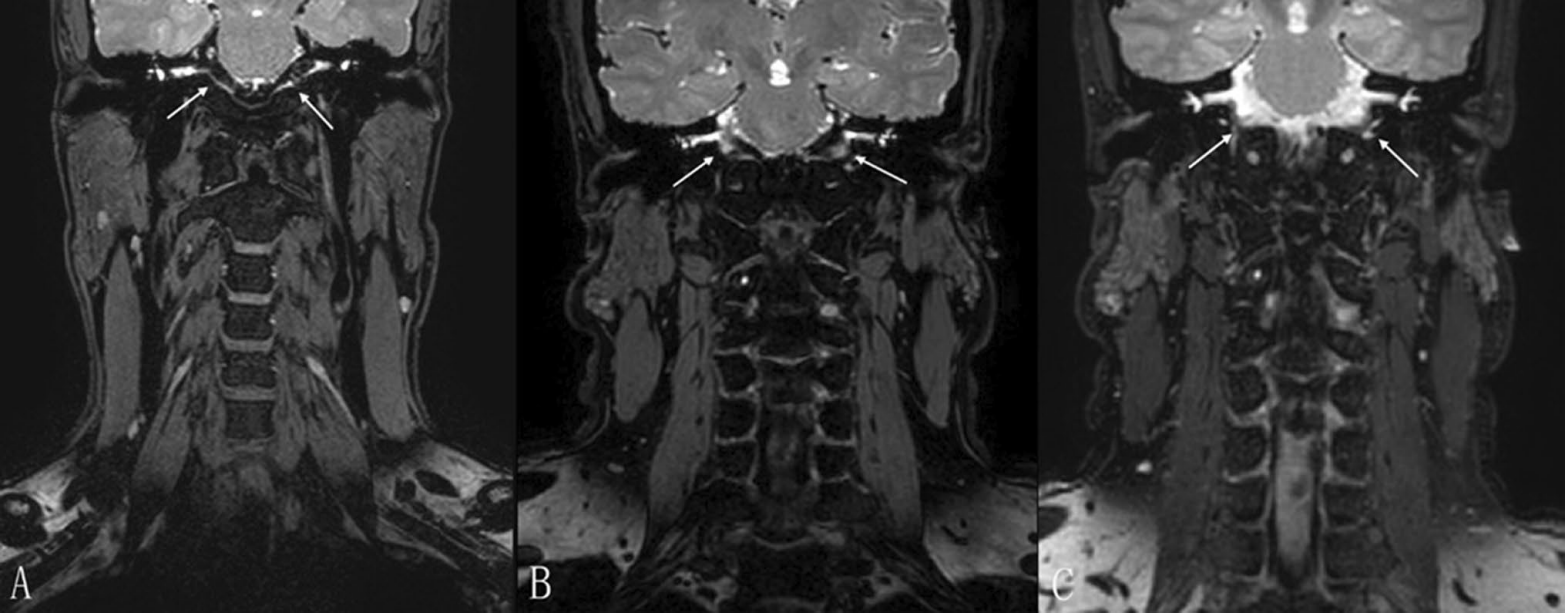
Examples of different grading scales of flow suppression in the cerebellopontine angle region (arrow). (A) Most flow suppression (grade 3) presented on 3D T2-weighted fast field echo (3D_T2_FFE). (B) Partial flow suppression (grade 2) presented on 3D_T2_FFE. (C) No flow suppression (grade 1) presented on 3D_T2_SPACE.
